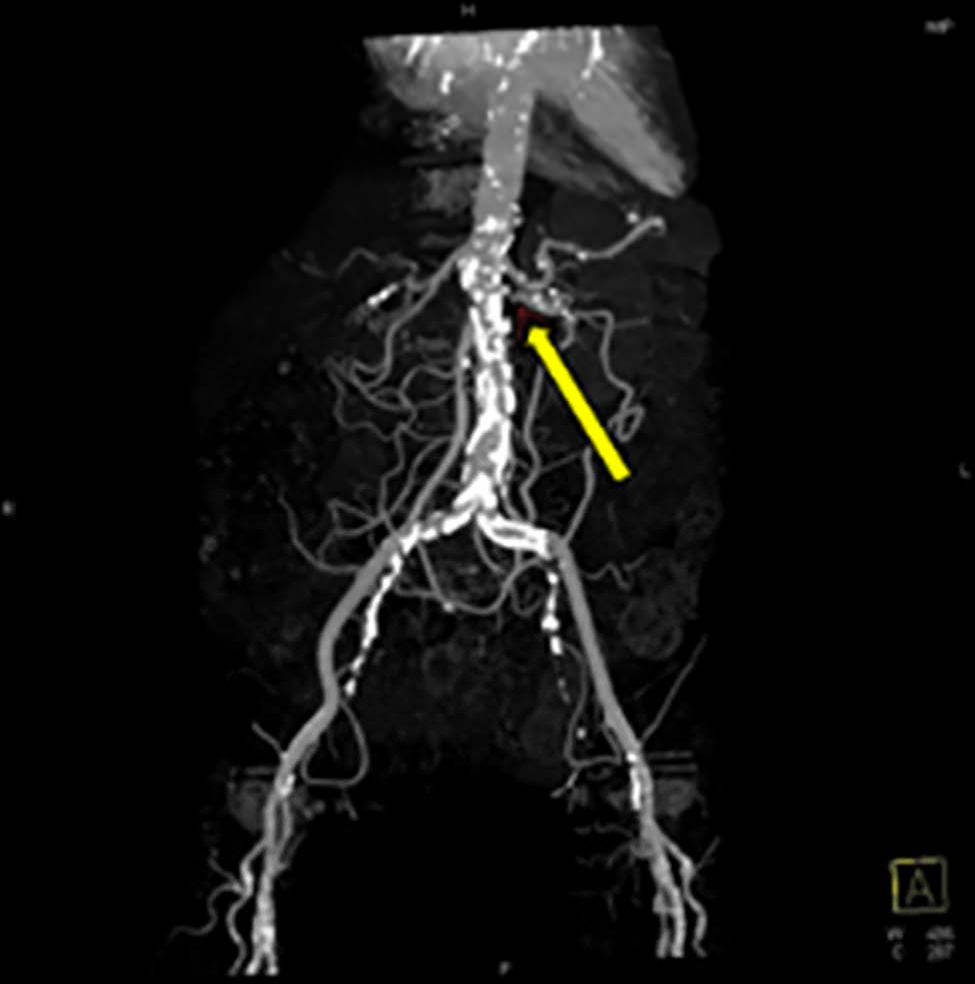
Computed tomography angiography reveals severe stenosis at the left renal artery orifice (yellow arrow).
